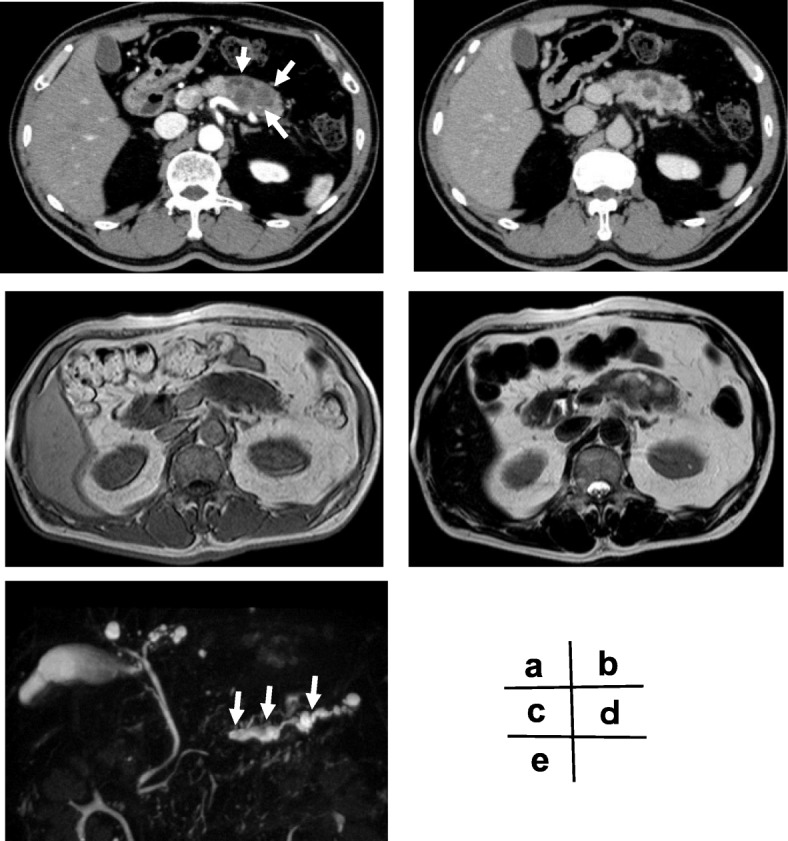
Contrast-enhanced CT scan (early phase) showed a low-density mass measuring 5 cm in diameter in the pancreatic body (arrows) (a). Contrast-enhanced CT scan (late phase) (b). MRI showed a tumor in the pancreatic body showing low intensity on T1-weighted images (c) and relatively high intensity on T2-weighted images (d). MRCP revealed a dilation of the distal main pancreatic duct (arrows) (e)
